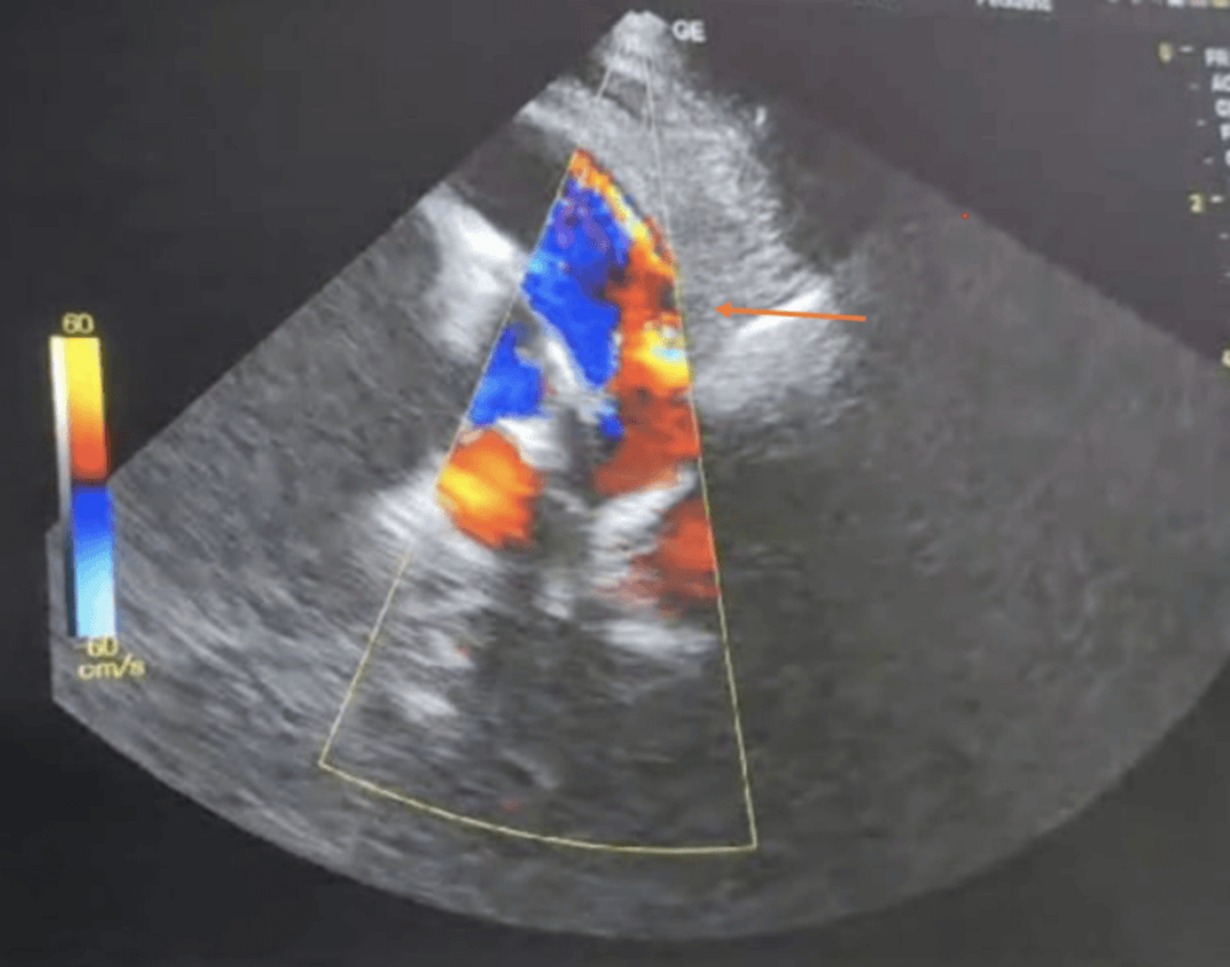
Echocardiography shows patent ductus arteriosus (PDA) in the parasternal short axis view
